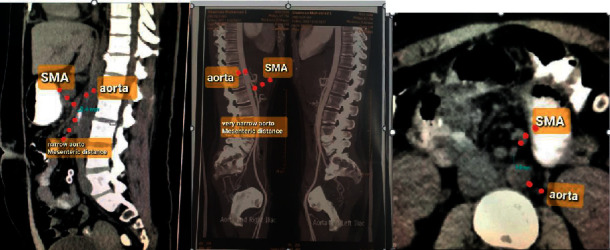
CT sagittal and coronal views showing narrow aortomesenteric distance.
